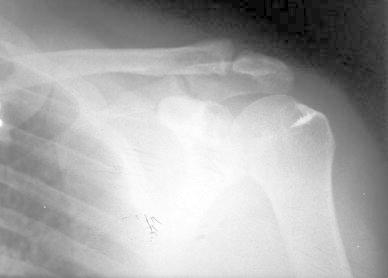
After rotator cuff repair roentgenogram
